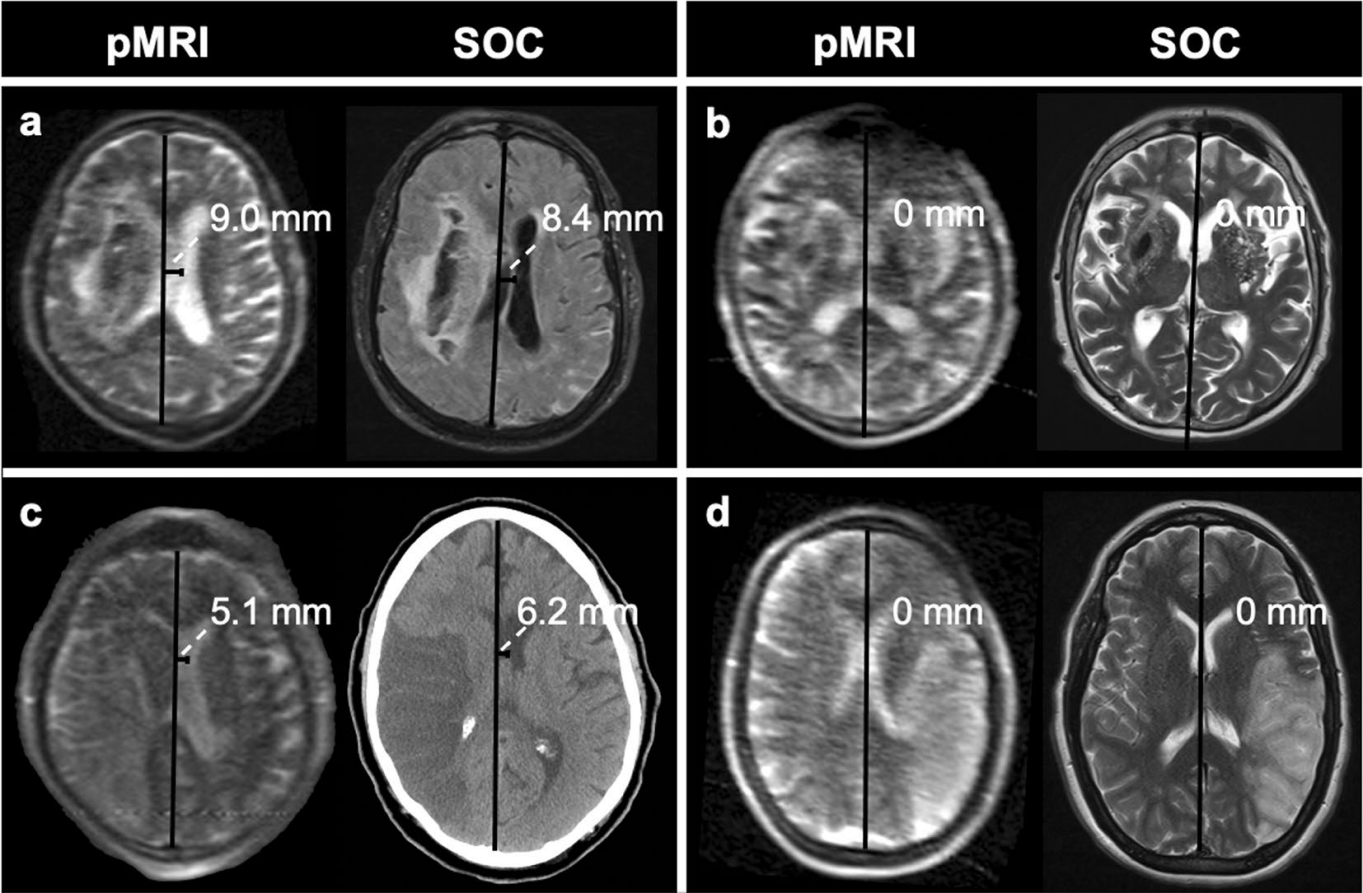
Example midline shift measurements on portable MRI (pMRI) and standard-of-care (SOC) imaging exams. (a) 81-year-old male with right intracerebral hemorrhage. Midline shift was measured to be 9.0 mm and 8.4 mm on the pMRI T2-weighted (T2W) and standard-of-care (SOC) MRI fluid-attenuated inversion recovery (FLAIR) images, respectively. (b) 43-year-old male with right intracerebral hemorrhage. No midline shift was measured on either pMRI T2W or SOC MRI T2W exams. (c) 71-year-old male with right M1 occlusion. Midline shift was measured to be 5.1 mm and 6.2 mm on the pMRI T2W and SOC CT images, respectively. (d) 44-year-old female with left M2 occlusion. No midline shift was measured on either pMRI T2W or SOC MRI T2W exams. Figure created using: Microsoft PowerPoint, Version 16.52, https://www.microsoft.com/en-us/microsoft-365/powerpoint.
